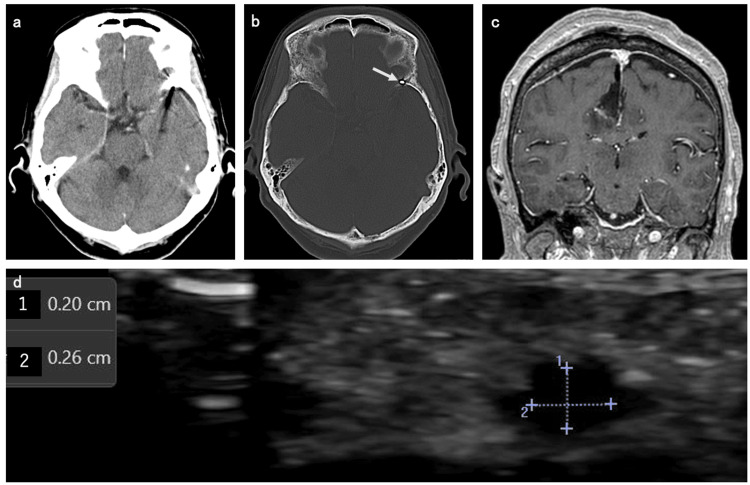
Postprocedural findings a, b: Postembolization computed tomography scans revealed no complications, including epidural hematoma, and embolized coils (white arrow). c: Postoperative contrast magnetic resonance imaging displays nearly total resection of the meningioma. d: No left radial artery occlusion at the puncture site confirmed using ultrasound examination.
